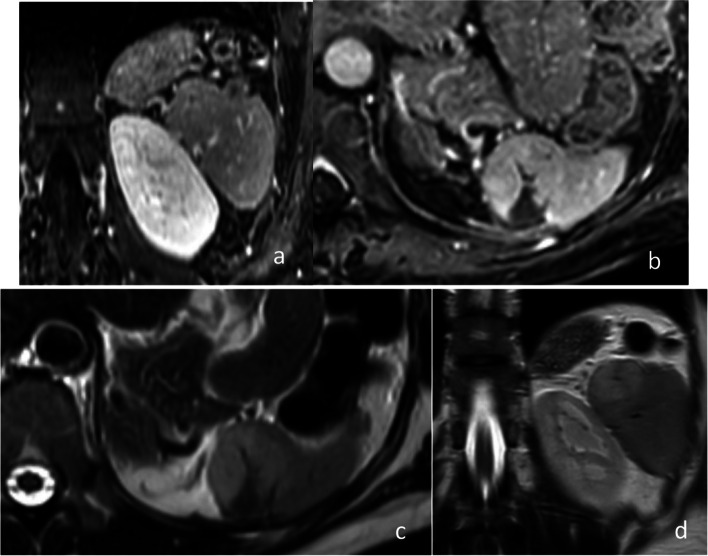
MRI examination 2 years post-embolization. a Normal spleen parenchyma (Coronal T1-Gd-weighted). b The known hemangioma on the upper spleen pole is now measured (3 × 2) cm (Axial T1-Gd-weighted). The lesion appears with moderate signal on axial (c) and coronal (d) T2-weighted images. A triangular area of post-embolization infarction is recognized (b, c)
